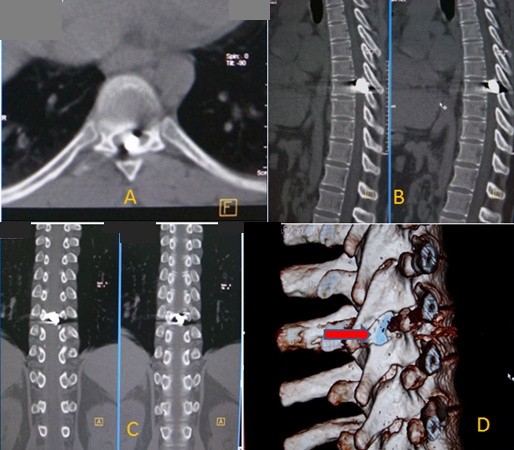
Imaging studies of the bullet injury. (A) Axial thoracic computed tomography (CT) image at the T8 level demonstrating the bullet nucleus in the canal without any bone destruction. Metallic artifacts can also be seen. (B) Two-dimensional sagittal reconstruction image, with the bullet nucleus in the mid spinal cord without bone destruction. Metallic artifacts can also be seen. (C) Two-dimensional coronal reconstruction CT image, with the bullet nucleus in the mid spinal cord without bone destruction. Metallic artifacts can also be seen. (D) Three-dimensional reconstruction CT image of the bullet in the intra-canalicular canal with no bone destruction. In this image the bullet setting can clearly be seen.
